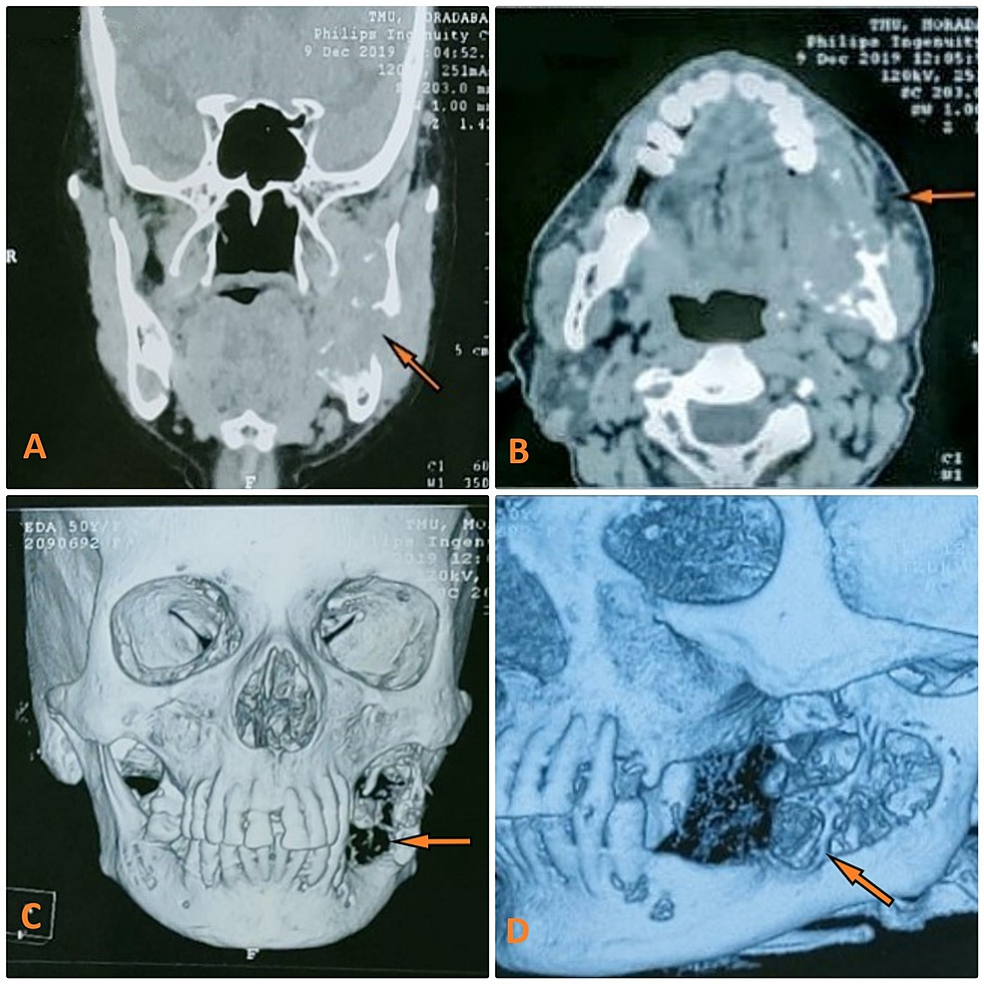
Computed tomography scans revealing aggressive bony resorption of the lower left jaw starting from premolar region and extending well into the mandibular ramus. (A) Coronal view. (B) Axial view. (C) Three-dimensional reconstruction frontal view. (D) Three-dimensional reconstruction lateral view.
